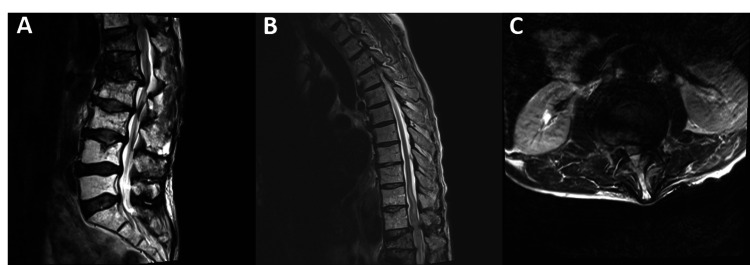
Sagittal (A & B) and axial (C) pre-operative MRI show severe compression at the level of T12 and L1.
